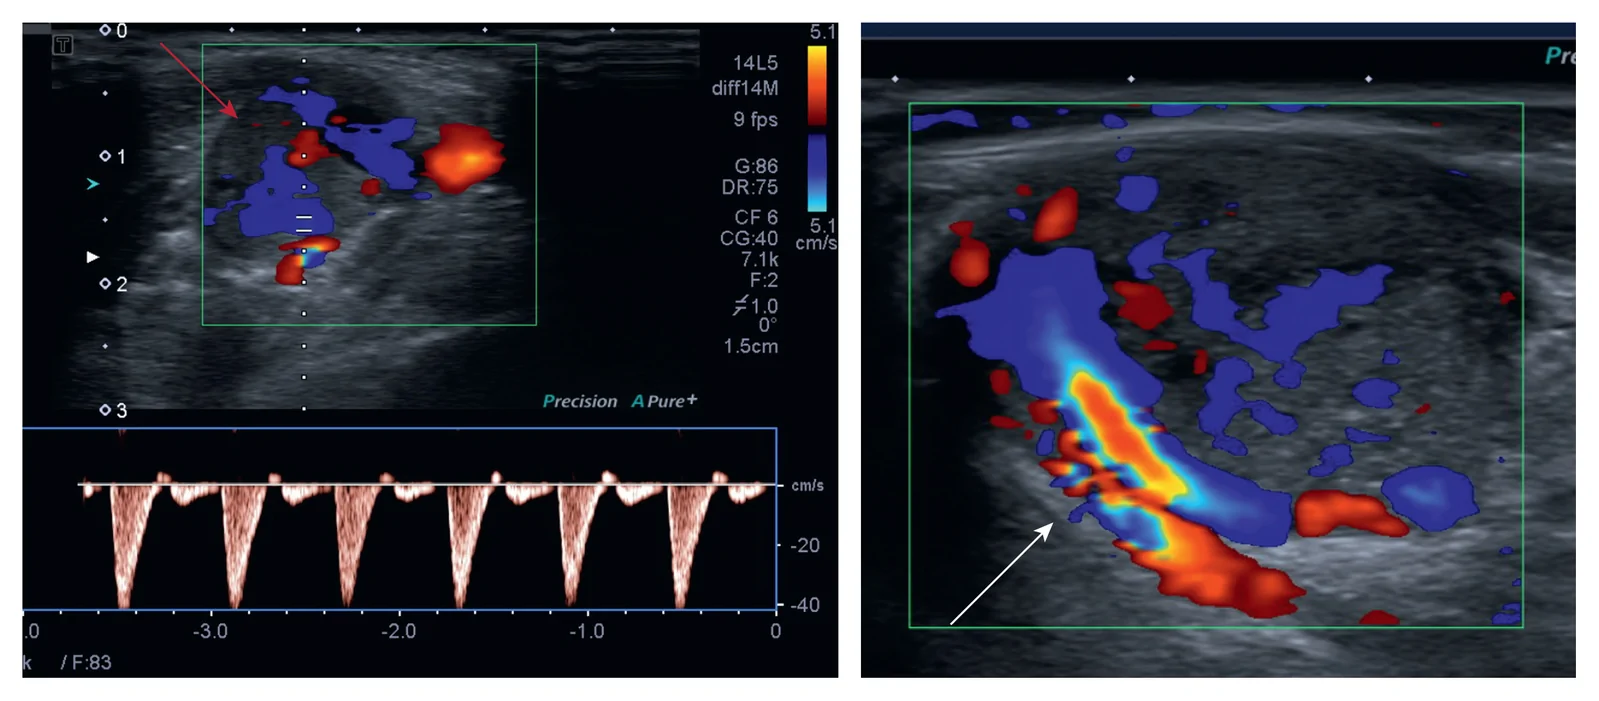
Ultrasound image showing a well defined, heterogeneous solid mass in the left antecubital fossa, with internal vascularity (red arrow) and an adjacent tubular structure suggestive of venous communication (white arrow).
